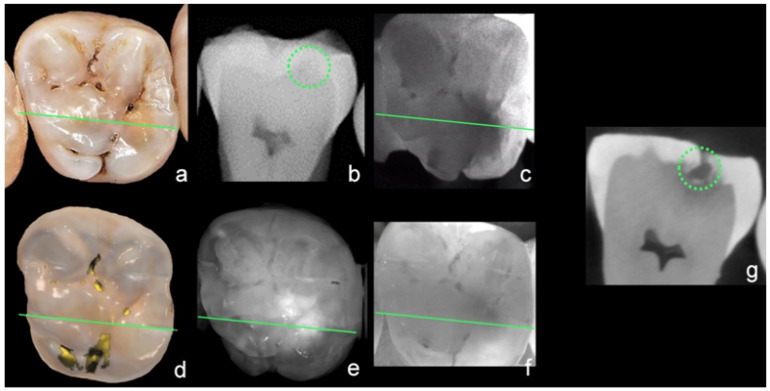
Example of all investigated caries diagnostic methods: visual examination (a), bitewing radiography (b), Diagnocam (c), Trios 4 (d), iTero Element 5D (e), Planmeca Emerald S (f), and µ-CT reference (g); green line shows plane of µ-CT, green circle marks caries lesion in sectional image.
